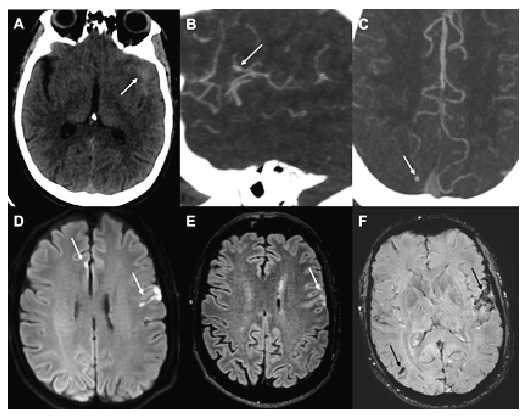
Head computed tomography (CT) showing a thin subarachnoid hemorrhage in the left Sylvian fissure (arrow in A). CT angiography demonstrating a small fusiform dilation in an opercular branch of the left middle cerebral artery (arrow in B) and a punctiform enhancement in the right superior parietal lobule (arrow in C), both of which were suggestive of mycotic aneurysms. Brain diffusion-weighted magnetic resonance imaging and FLAIR revealed multiple cortical infarcts (white arrows in D and E), consistent with embolic stroke. Susceptibility-weighted Imaging also showed microbleeds in the left frontal and right parietal sulci (arrows in F).
